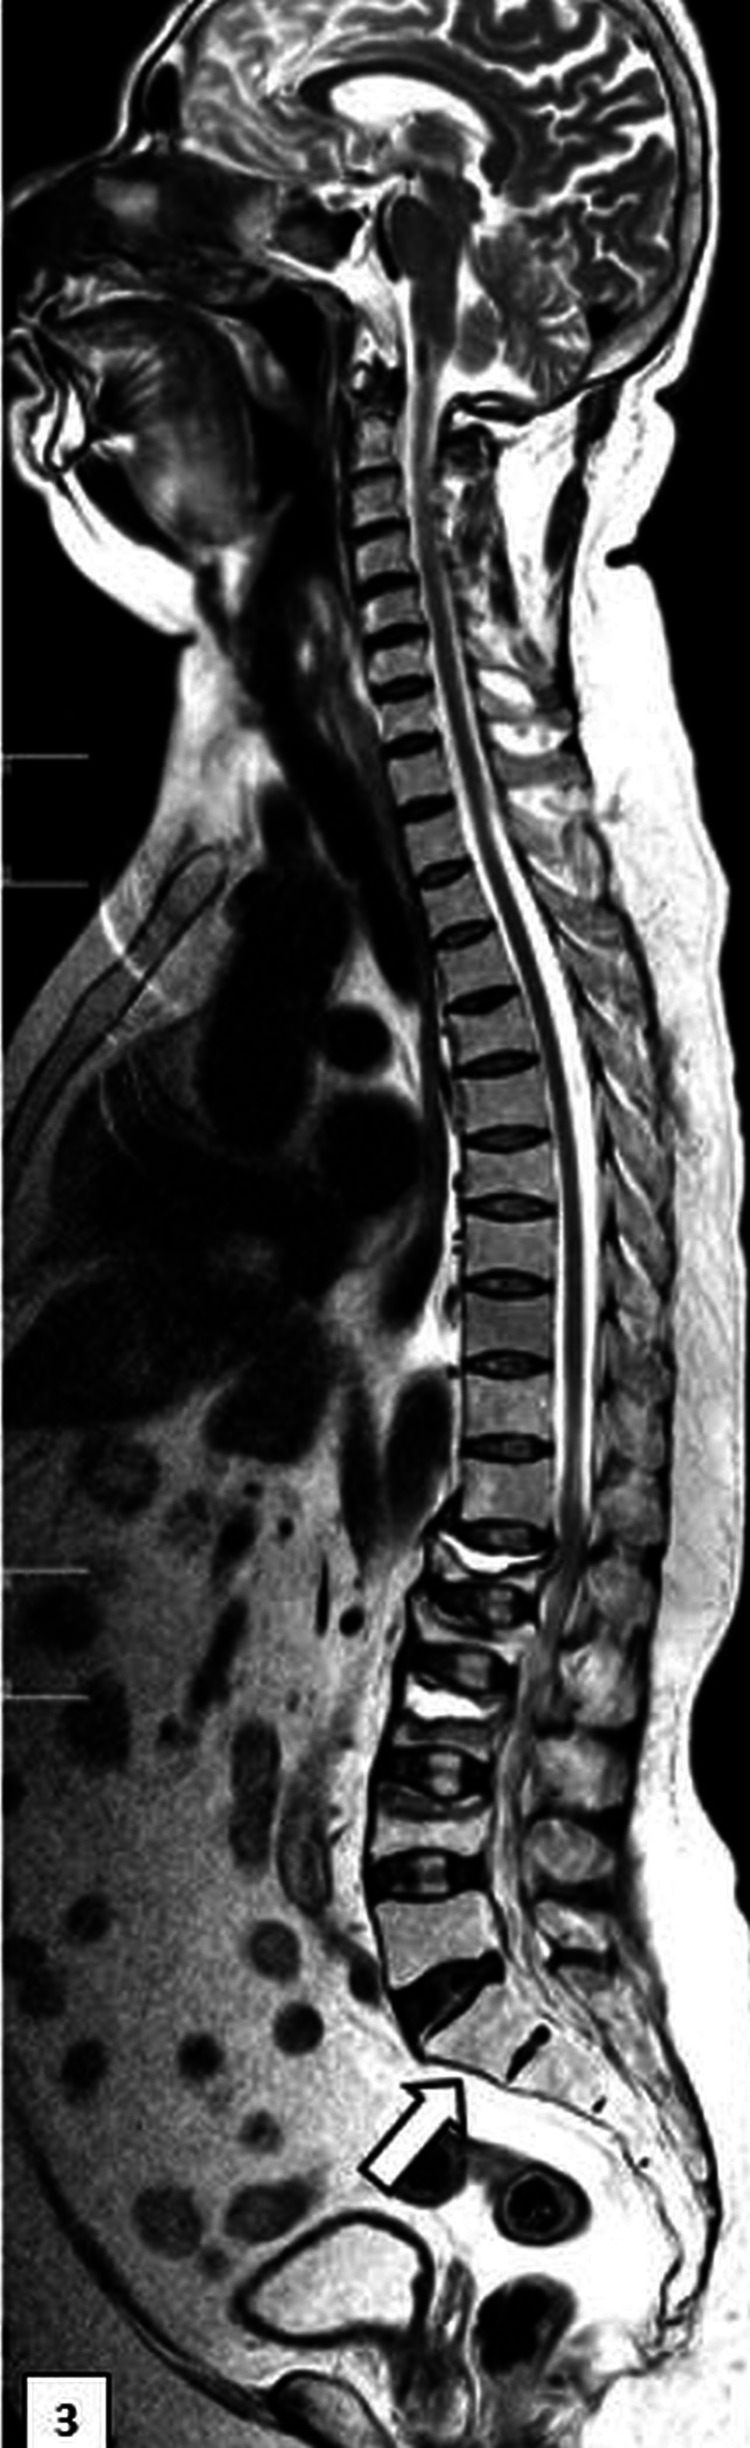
Magnetic resonance image of the whole spine Loss of normal curvature with sacralization of the lumbar vertebrae is shown.
